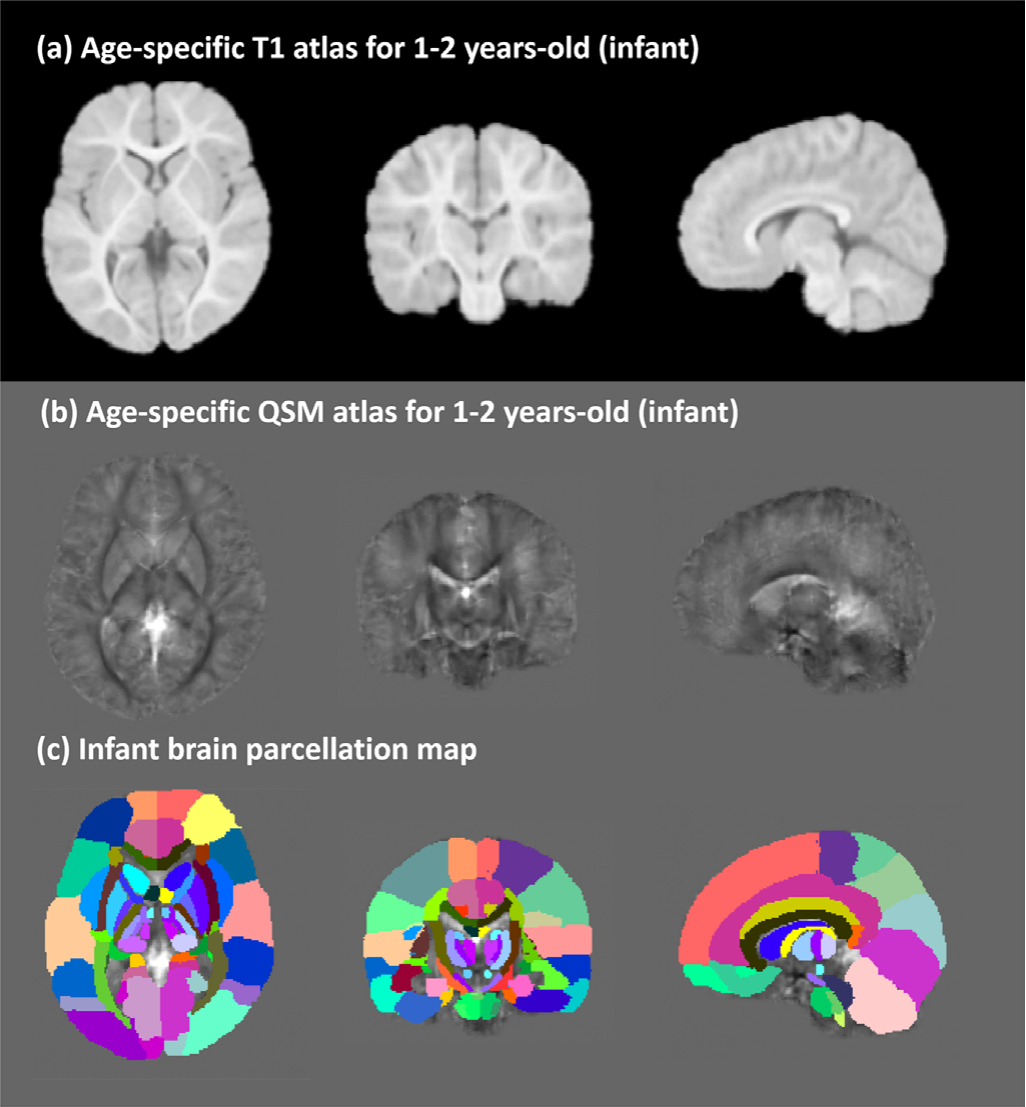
Performance of infant brain age-specific QSM atlas. (a) The T1-weighted infant atlas. (b) The QSM infant atlas. (c) The “infant whole brain QSM parcellation map”.
